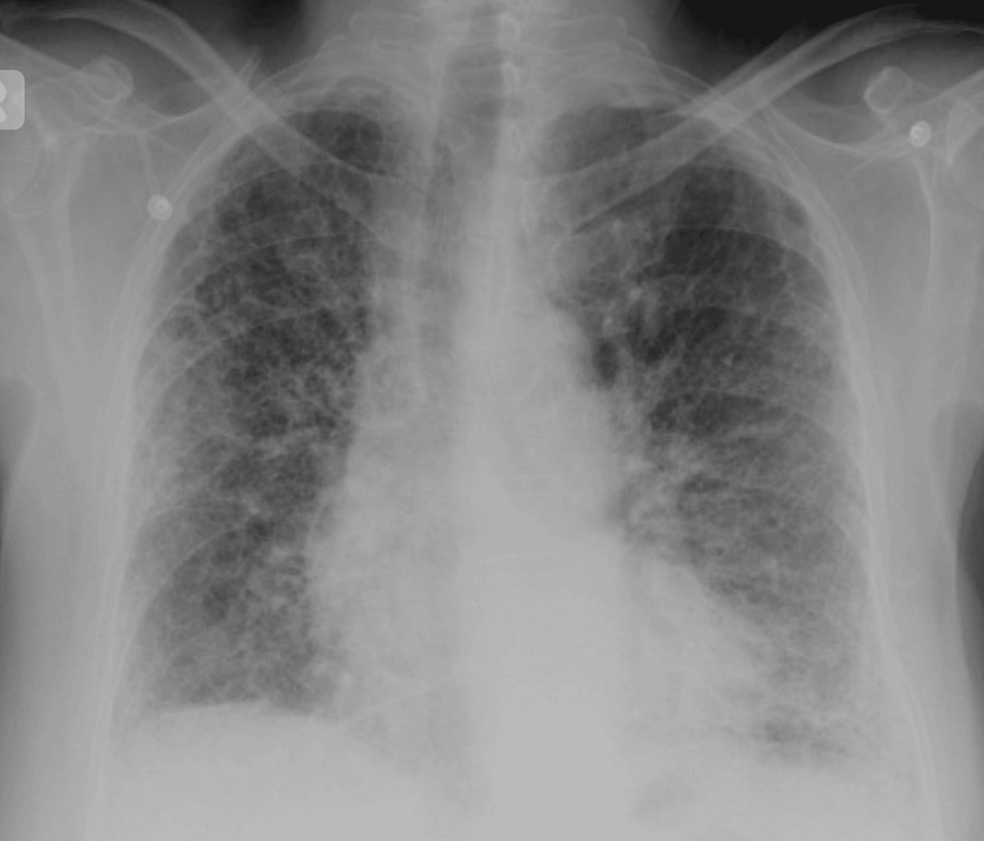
The chest X-ray showed bilateral pulmonary opacities suggestive of COVID-19 pneumonia with an underlying pulmonary fibrosis
